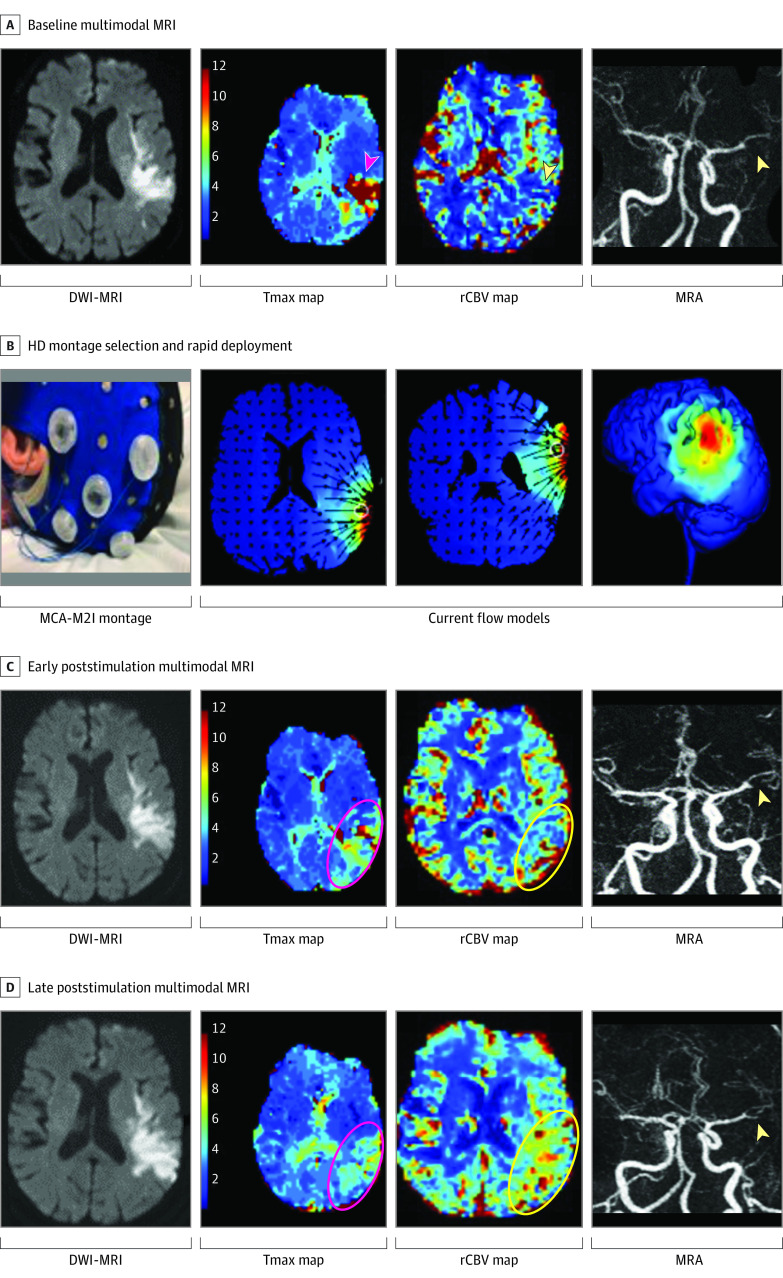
Second Exemplar of a Transcranial Electrical Stimulation in Stroke Early After Onset Clinical Trial Patient Patient 2 was a female in her 70s with acute ischemic stroke (AIS) due to left middle cerebral artery (MCA) inferior division occlusion. She received 20 minutes of 1 milliamp (mA) high-definition (HD) cathodal transcranial direct current stimulation to the left parieto-temporal region. Early alleviation of hypoperfusion region, enhancement of relative cerebral blood volume, and recanalization were observed on the stimulated side. A, Acute infarction of the left parieto-temporal region (diffusion-weighted image [DWI] hyperintensity); hypoperfusion of the parieto-temporal region (time-to-maximum [Tmax] >6 seconds); normal to low left parieto-temporal relative cerebral blood volume (rCBV); left MCA-M2 occlusion. B, A selection of MCA-inferior division HD montage with current model predicting the e-field concentration over the ischemic region. C and D, Evolving left parieto-temporal acute infarct (DWI hyperintensities); early and sustained alleviation of hypoperfusion; early and sustained rCBV enhancement of the stimulated site; early and lasting left MCA-M2 partial recanalization. MRI indicates magnetic resonance imaging.
